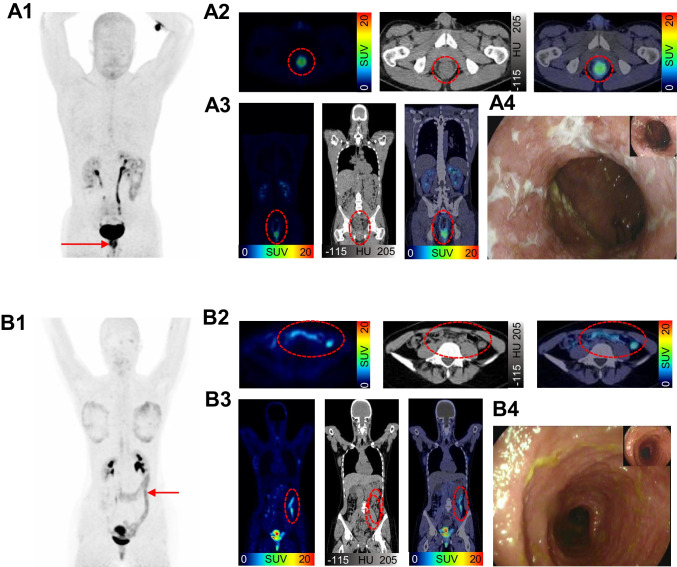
Examples of static ^68^Gallium- fibroblast activation protein inhibitor-based radiopharmaceuticals-46-positron emission tomography/computed tomography (^68^Ga-FAPI-46-PET/CT) imaging of 32-year old male with Crohn´s disease (CD) (A) and of 18-year old female with ulcerative colitis (UC) (B). 1 ^68^Ga-FAPI-46-PET with maximum intensity projection (MIP) 2 Transversal ^68^Ga-FAPI-46-PET/CT and fused ^68^Ga-FAPI-46-PET/CT 3 Coronal ^68^Ga-FAPI-46-PET/CT and fused ^68^Ga-FAPI-46-PET/CT 4 Example of colonoscopy. The FAPI-positive inflammatory bowel disease (IBD) lesion of CD in the rectum (see arrow in A1) is seen with a high uptake (SUVmax: 9.3) in reconstructed images (see red circle in A2 and A3) and corresponds to the endoscopic finding in A4. The example in B1 shows a long-standing FAPI-positive IBD lesion (SUVmax: 6.8) in the colon in UC, which is also visible on reconstructed ^68^Ga-FAPI-46-PET/CT in B2 and B3. Also this finding corresponds to the endoscopic reading
